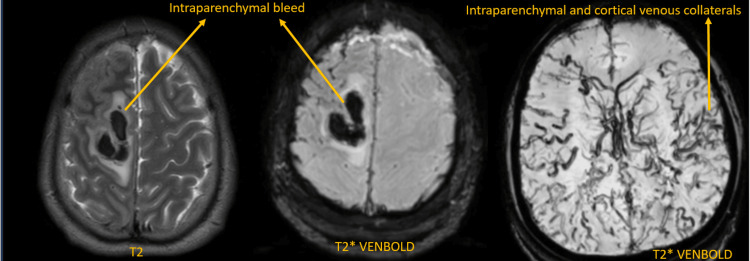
Follow-up MRI in T2W and T2* axial sections shows intraparenchymal bleed in the right frontal lobe. T2W: T2-weighted image.
